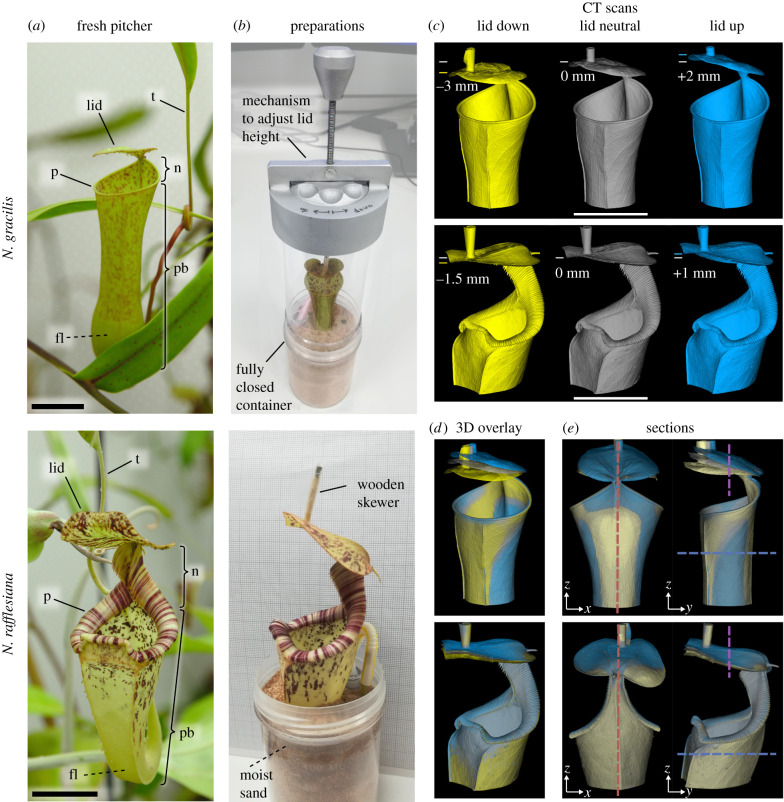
Experimental procedure to visualize pitcher deformation associated with lid displacement (scale bars = 25 mm). (*a*) Pitchers of *N. gracilis* (top) and *N. rafflesiana* (bottom); t, tendril; p, peristome; n, neck; pb, pitcher body; fl, pitcher fluid. (*b*) For µ-CT scanning, the pitcher was embedded in moist sand within a sealed container. The position of the pitcher lid could be adjusted via a device in the container lid. (*c*) Each pitcher was scanned with three different lid positions: neutral (grey), downward (yellow) and upward (blue), and (*d*) the resulting three-dimensional models were overlaid. (*e*) Longitudinal sections through the dorsal spine (red) and cross-sections through the pitcher body and neck (blue) and through the lid (purple) were used to analyze the deformation.
